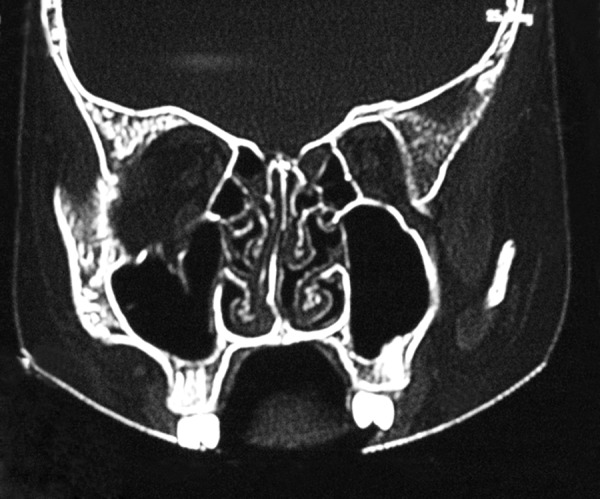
Left floor of orbit fracture in CT scan
